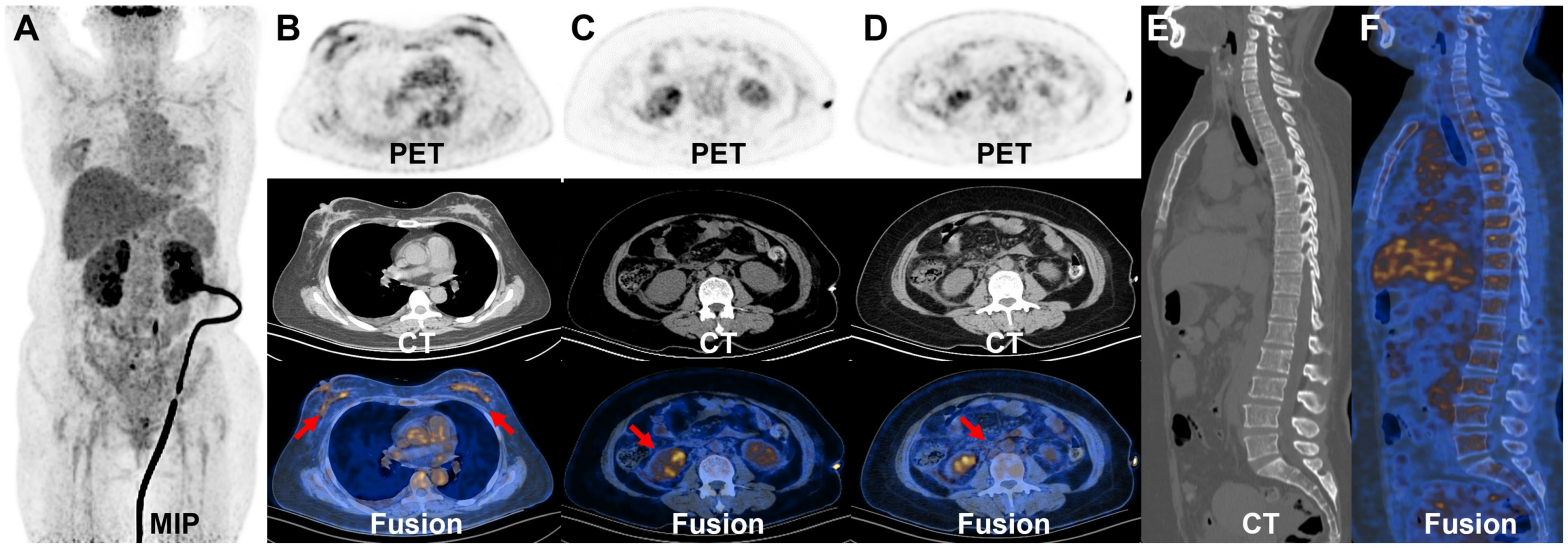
^18^F-FDG PET/CT images. The MIP demonstrated patchy metabolic elevation on both sides of the mammary glands, as well as increased volume in both kidneys, accompanied by a diffusely uneven elevated metabolic lesion (A). The bilateral dense breast tissue shows diffuse mild ^18^F-FDG uptake, with an SUVmax of approximately 4.7. There is a local thickening of the skin in both breasts, with an SUVmax of 2.3, more prominent on the right side (B). The transverse images show inhomogeneous ^18^F-FDG uptake in the bilaterally enlarged kidneys, with an SUVmax of approximately 8.2 (C). The transverse images show right perirenal fascia thickening with a significantly higher uptake of an SUVmax of approximately 3.3 (D). The sagittal images show the heterogeneous bone density elevation with radioactive distribution, SUVmax of approximately 2.9 (E,F).
